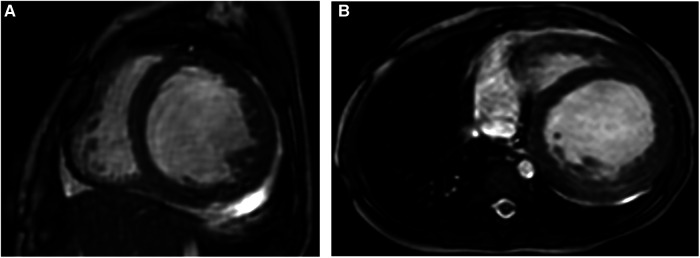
Representative MRI scans of a 1-year-old female patient with EFE who presented with severe heart failure and had PCR once due to the heart event. (A,B) Short-axis and axial FIESTA cine showing thickened endocardium with obviously increased and hypertrophied myocardial trabeculae. EFE, endocardial fibroelastosis.
